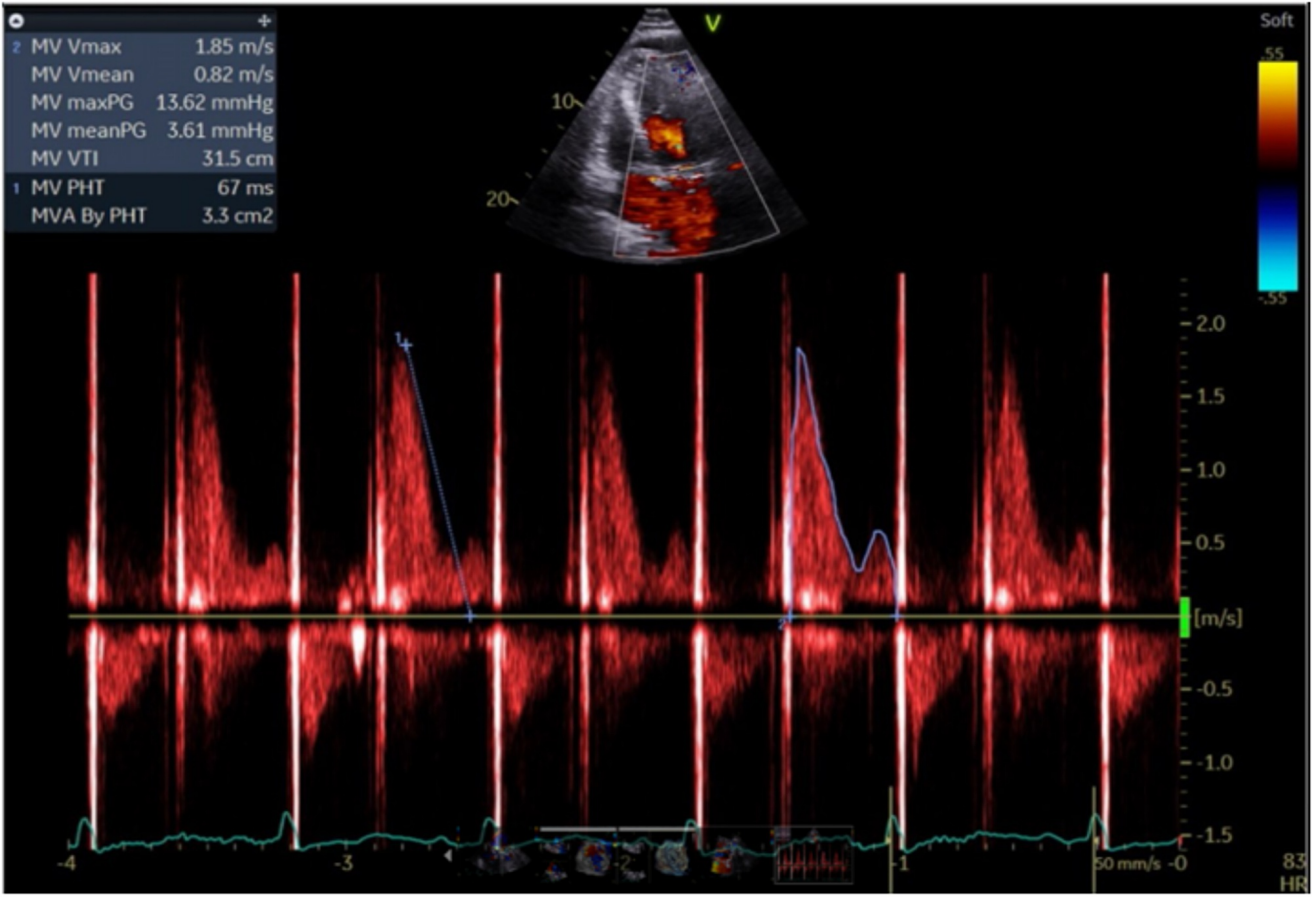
Mitral inflow velocity mapping on transthoracic echocardiogram without evidence of significant mitral regurgitation
